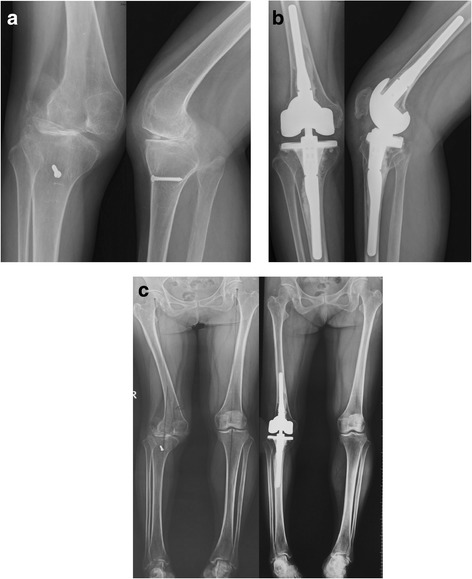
Implantation of CCK prosthesis in a 62-year-old female with post-traumatic arthritis with valgus deformity and significant instability of the right knee. a Pre-operative anteroposterior (AP) and lateral radiographs; she had undergone some type of procedure on her index knee. b Post-operative radiograph shows 3° of valgus and no radiolucent lines. c Comparison of pre-operative and post-operative long leg radiographs
